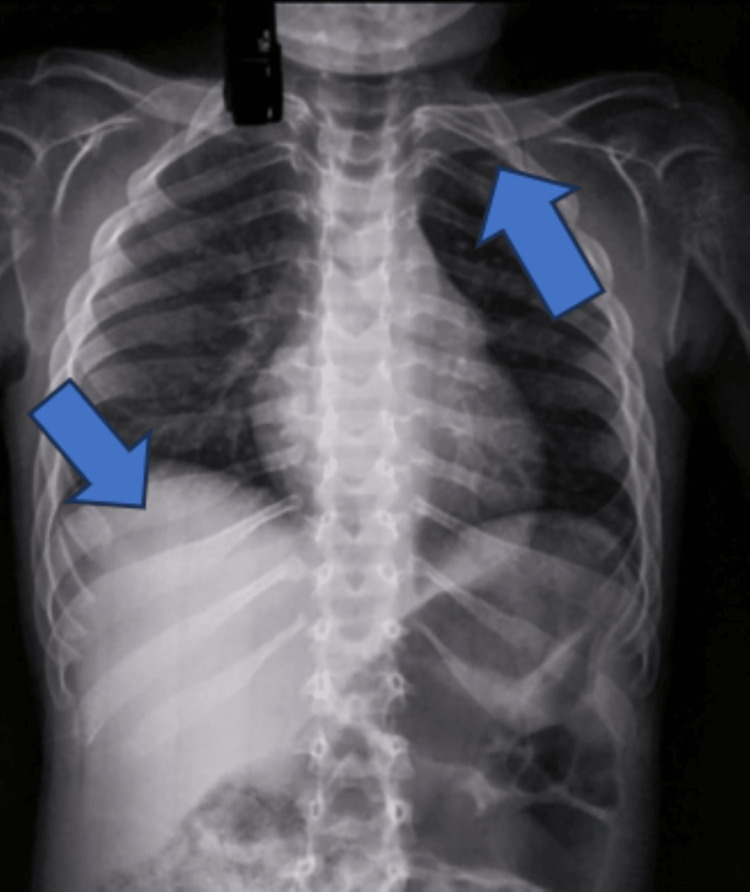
Chest X-ray findings (arrows) of the child showing oar-shaped ribs (9th to 12th ribs) and thickened clavicle.
